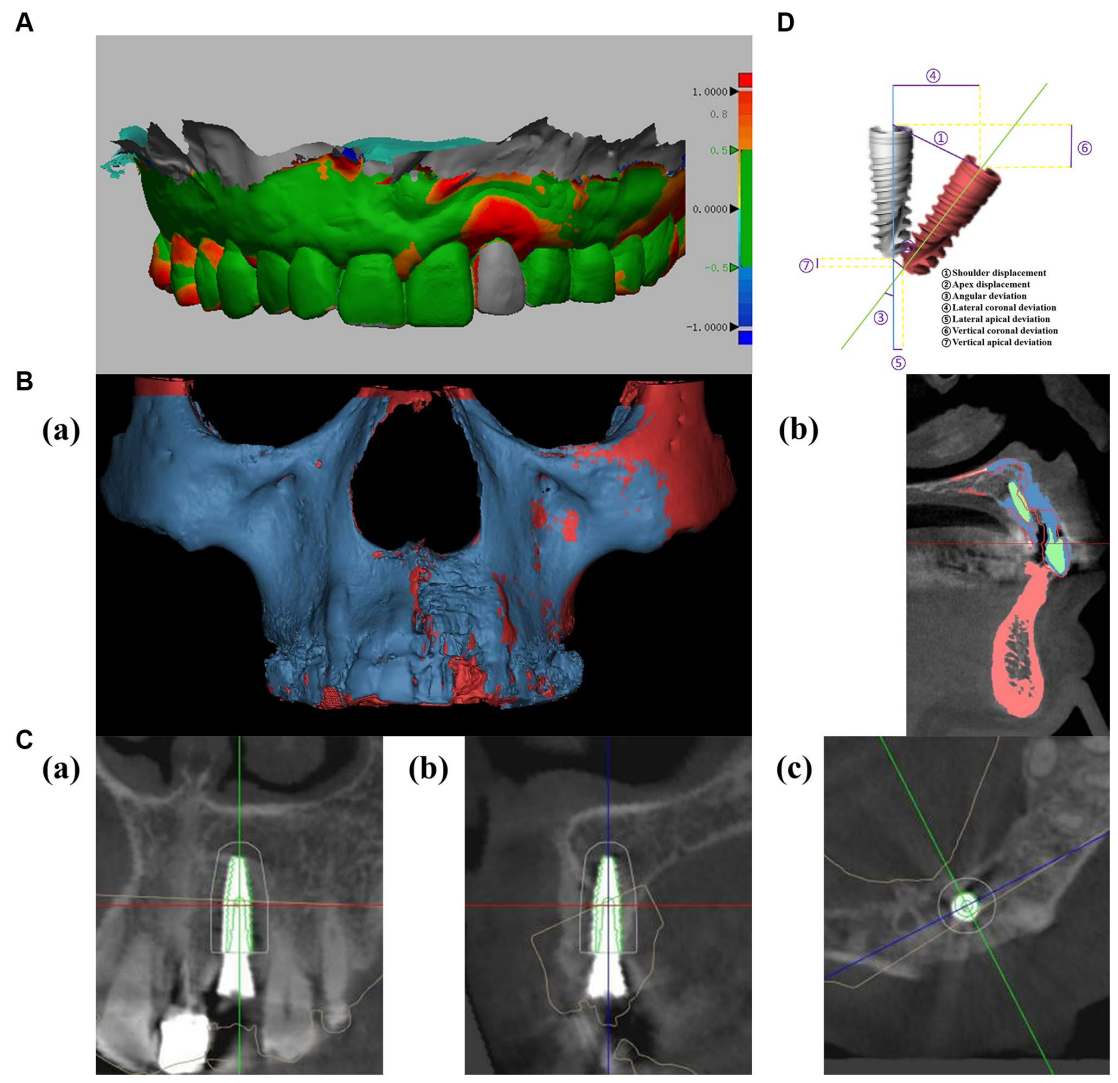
(A) Analysis of soft tissue recession using oral scanning data (soft tissue volumes were compared by comparing pre-and post-operative oral scan data, and color represented differences in gingival changes, which could be defined by the right scale). (B) Analysis of bone volume changes using CBCT (red represents preoperative bone tissue, blue represents postoperative bone tissue, and green represents implants and restorations). (C) Postoperative evaluation: comparing accuracy of preoperative planned implant position (green) with postoperative position. (D) Deviations of planned (gray) and actual position (red) of implant.
